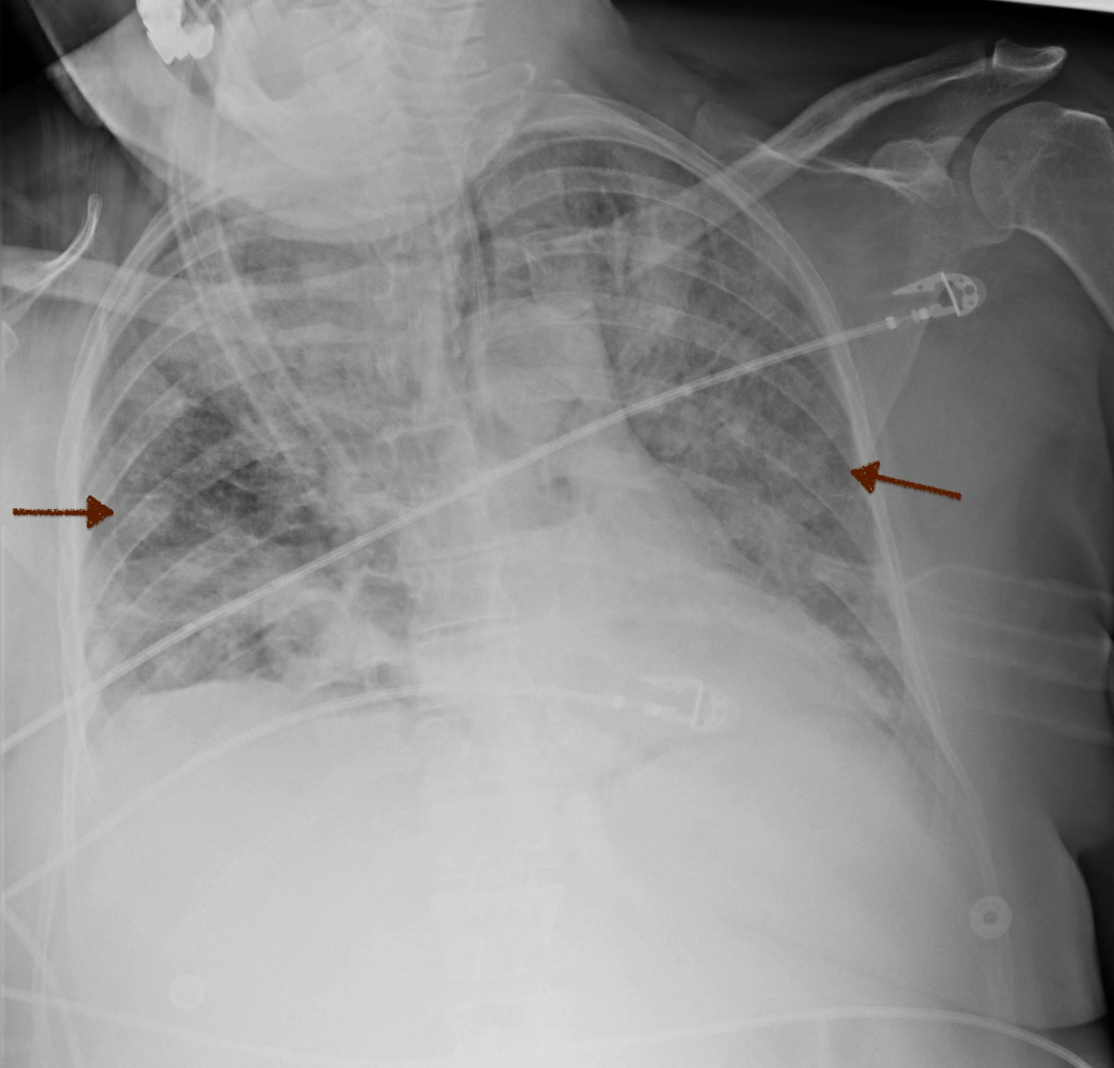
Chest X-Ray Diffuse bilateral airspace infiltrates postsurgery (red arrows).
